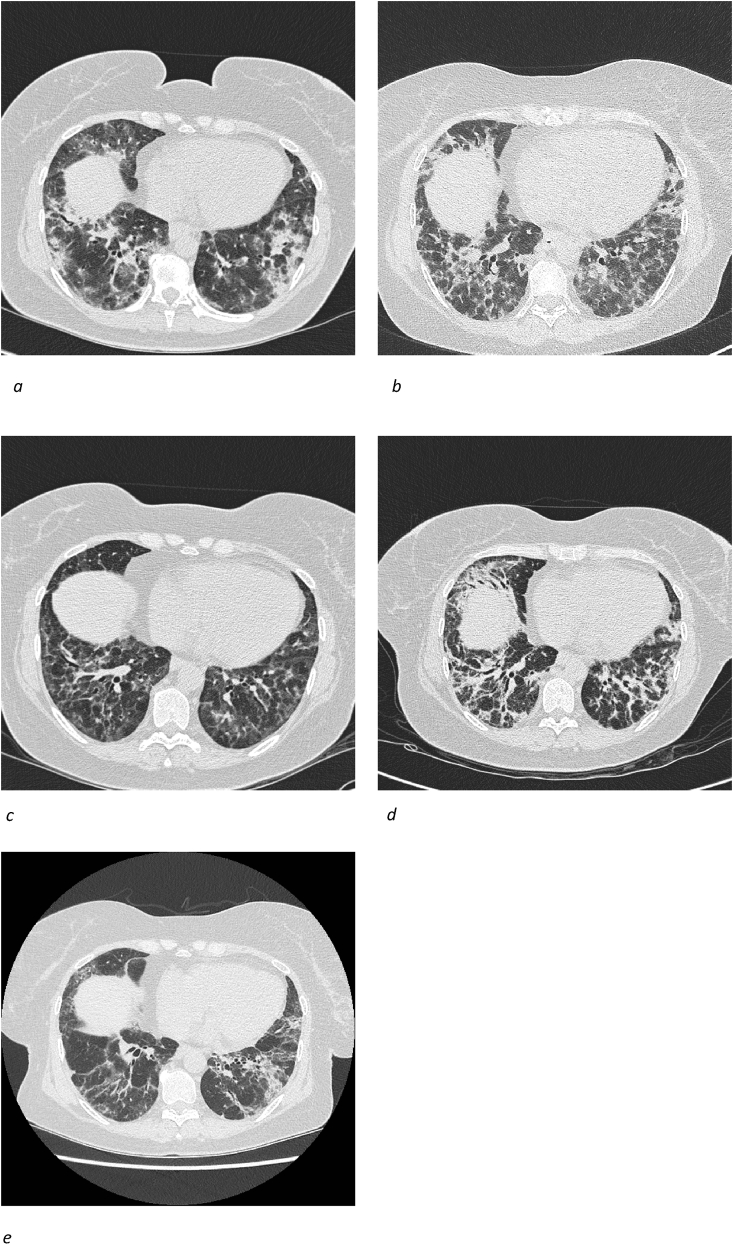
High resolution computed tomography (HRCT) of the lungs with interstitial changes during the treatment. Fig. 1a: *HRCT with interstitial lung disease with bibasilar predominance of peribronchial consolidations suspect of organizing pneumonia (OP) and non-specific interstitial pneumonia (NSIP);*Fig. 1b*: CT scan with progression of the interstitial findings;*Fig. 1c*: Significant regression of the interstitial changes after methylprednisolone;*Fig. 1d*: Basal reticulation, ground-glass opacities and perilobular consolidations consistent with OP and NSIP;*Fig. 1e*: HRCT with significant regression of the interstitial findings.*
